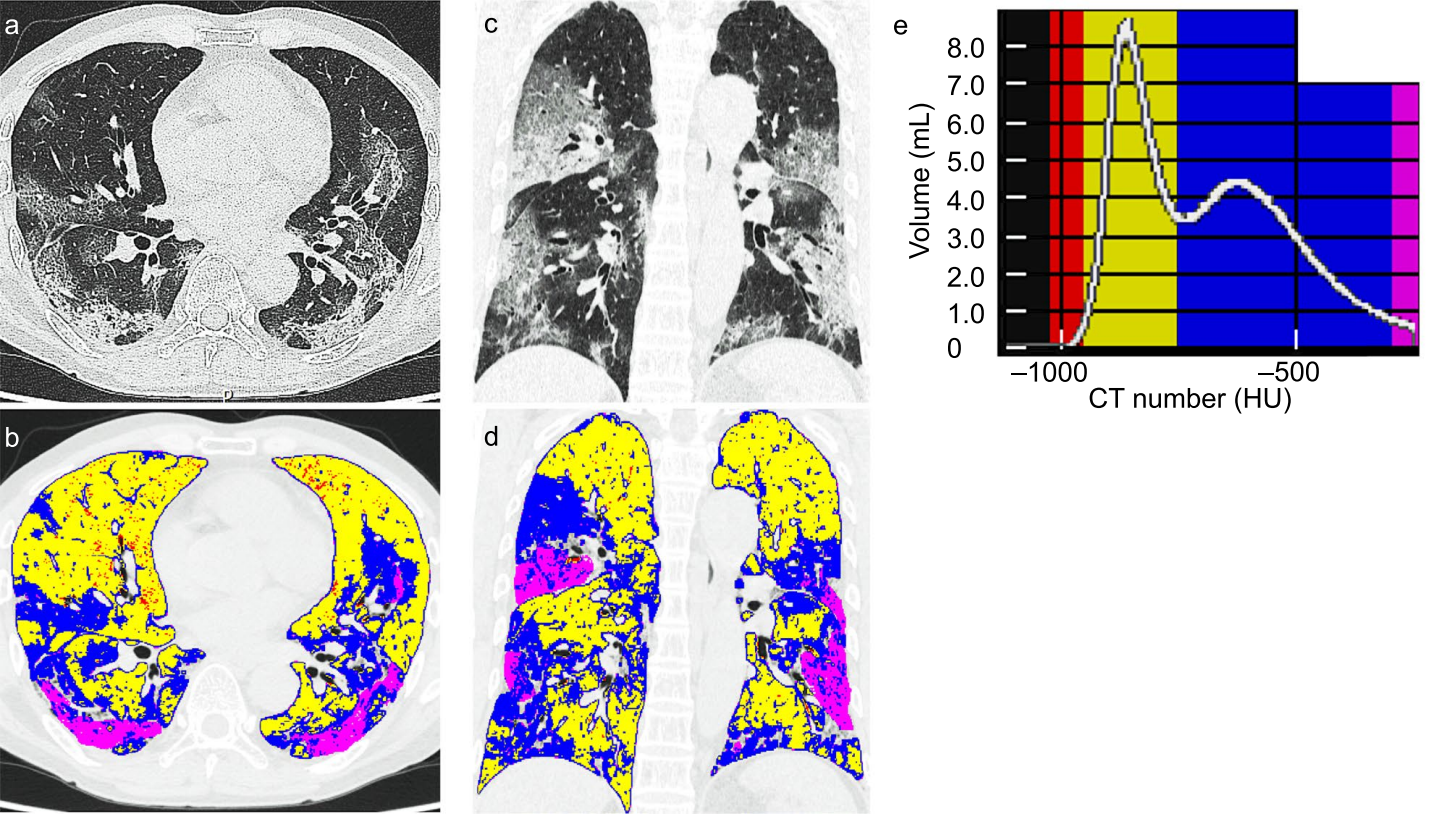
An example of the automated quantification of disease progression of COVID-19 pneumonia on CT. (a–d) CT findings of COVID-19 pneumonia by pre-setting a threshold value of Hounsfield Units and a color in order to categorize both lungs as emphysema (− 1024/− 950; red), healthy lung parenchyma (− 949/− 750; yellow), GGO (− 749/− 300; blue), or consolidation (− 299/40; violet). (a,b) Axial section. (c,d) coronal section. (b,d) Automated segmentation using an image processing tool. (e) Automated calculation of the volume and percentage of the impaired area, i.e. GGO and consolidation, relative to the whole lung. *COVID-19* coronavirus disease 2019, *GGO* ground-glass opacity, *HU* Hounsfield unit.
